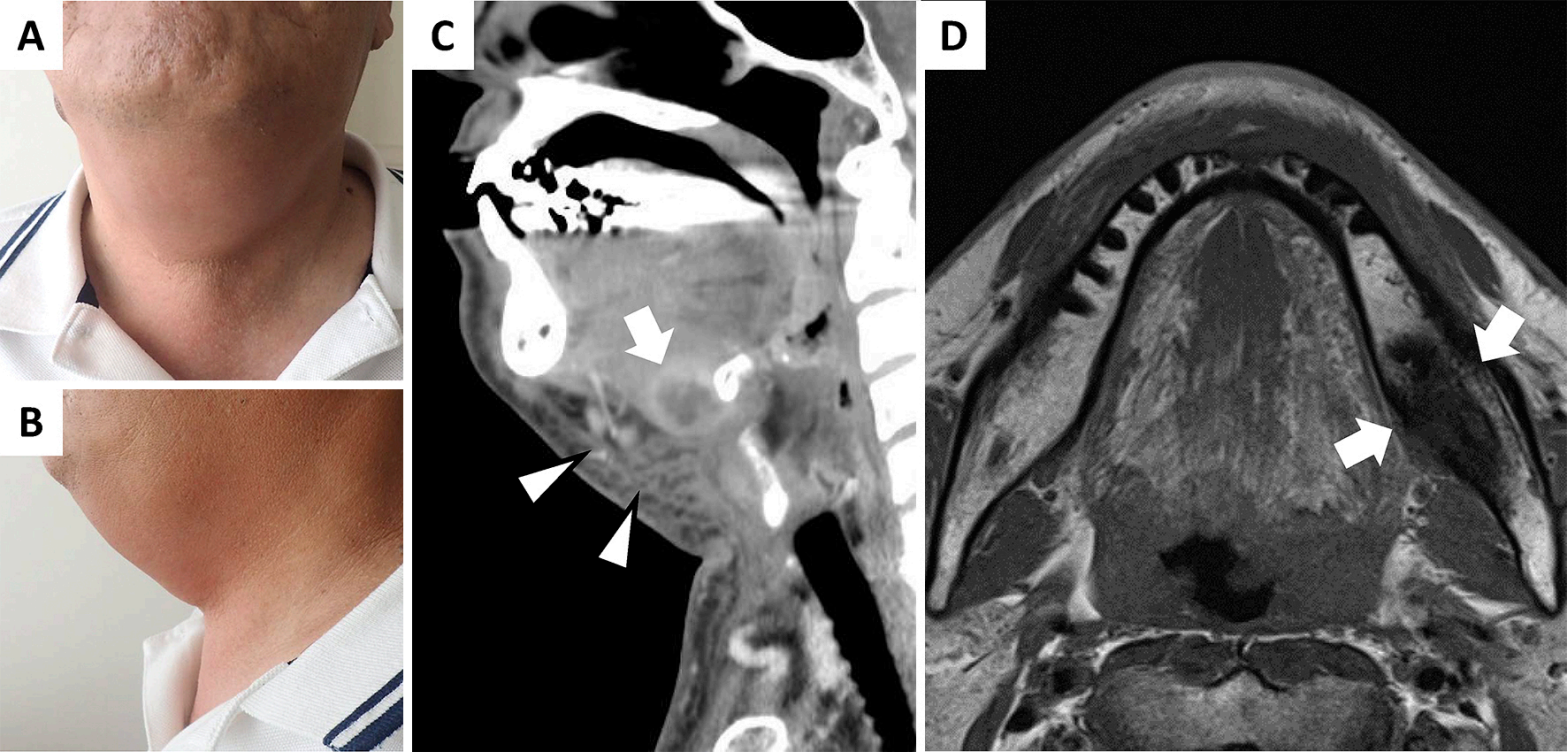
(A), (B) Physical examination revealed swelling and redness in the jaw. (C) Contrast-enhanced computed tomography (CT) demonstrated cellulitis in the patient’s jaw (*arrowheads*) and formation of an abscess (20-mm diameter) in front of the hyoid bone (*arrow*). (D) Magnetic resonance imaging (MRI) showed a low signal intensity lesion in the body of the left mandible on the T1-weighted image (*arrows*).
